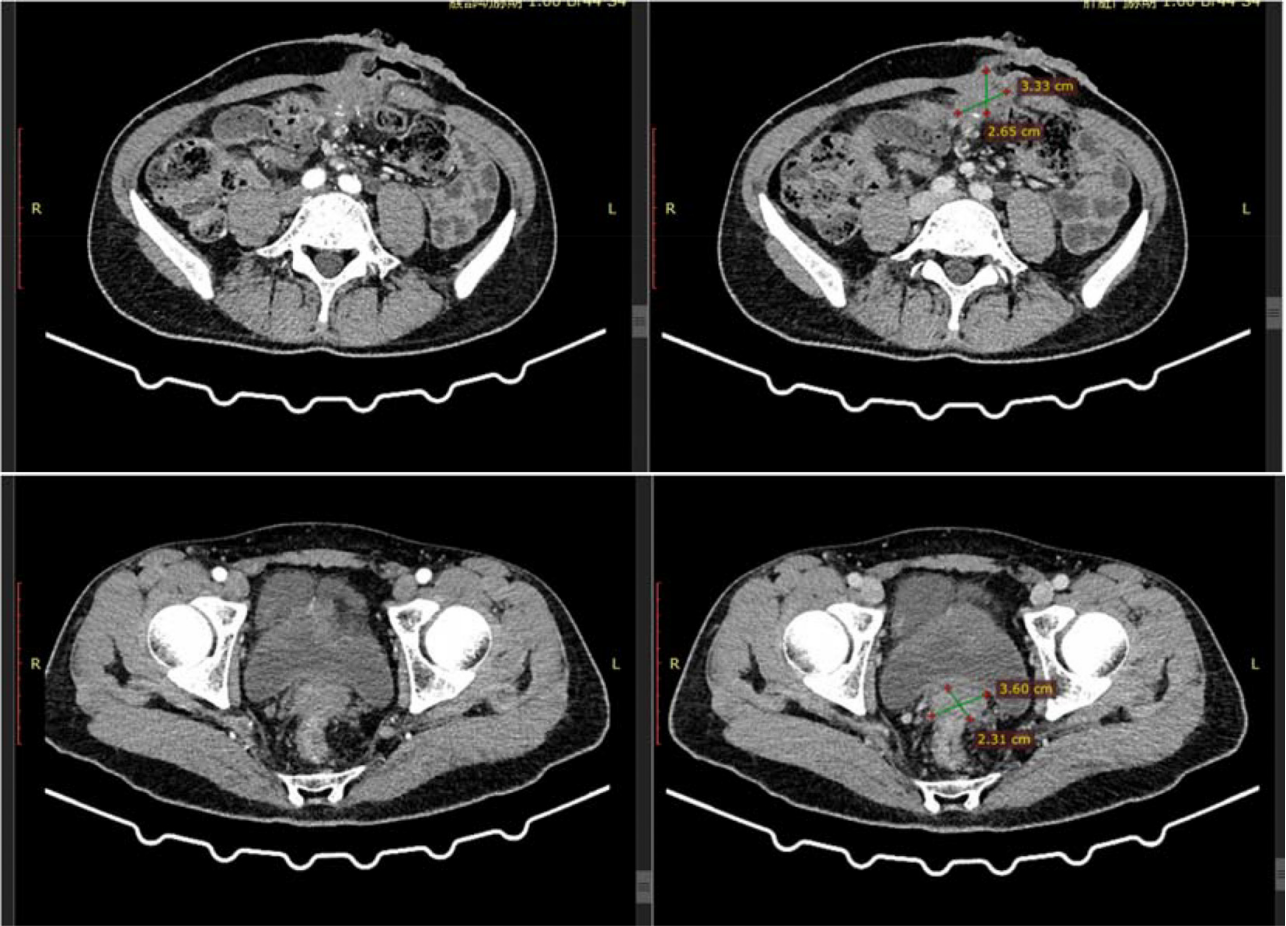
Abdominal CT in February 2023.
